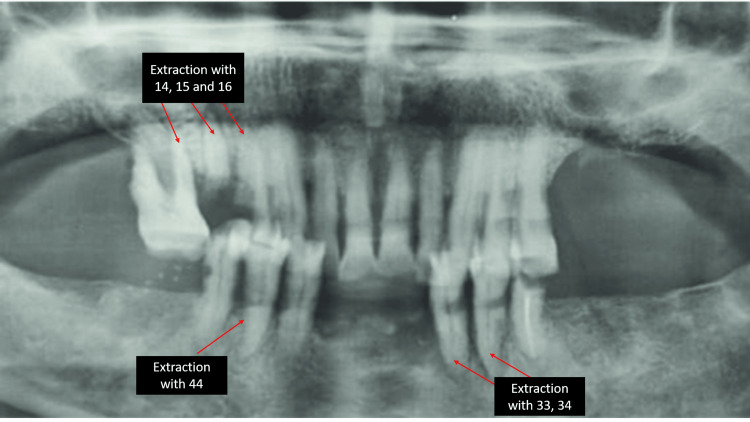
Radiographic examination Maxillary arch: extractions with 14, 15 and 16 Mandibular arch: extractions with 33, 34 and 44
